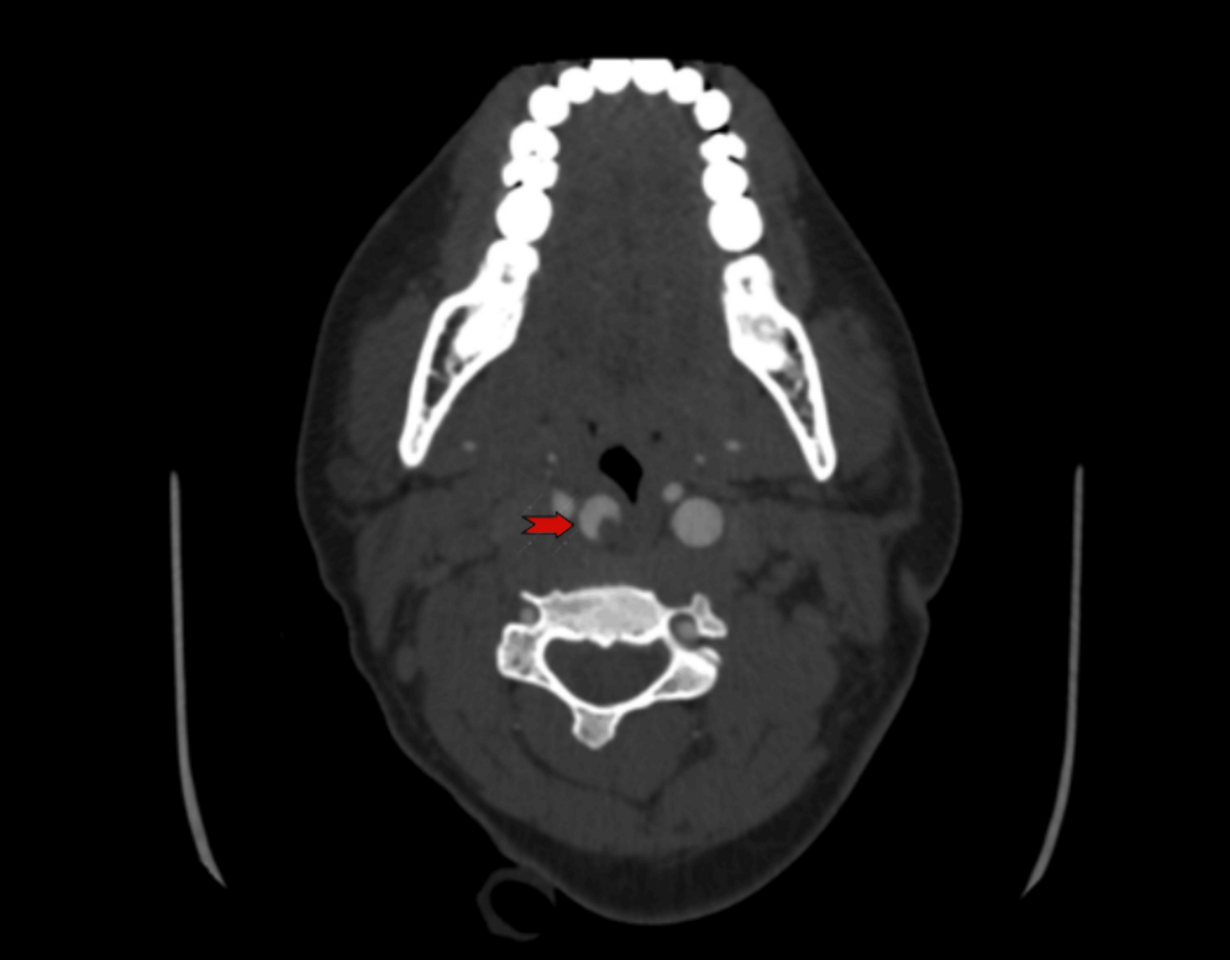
CT angiography (Axial view) Revealed acute occlusion at the right M1-M2 junction, with a soft thrombus at the origin of the right internal carotid artery causing 45% occlusion (red arrow).
